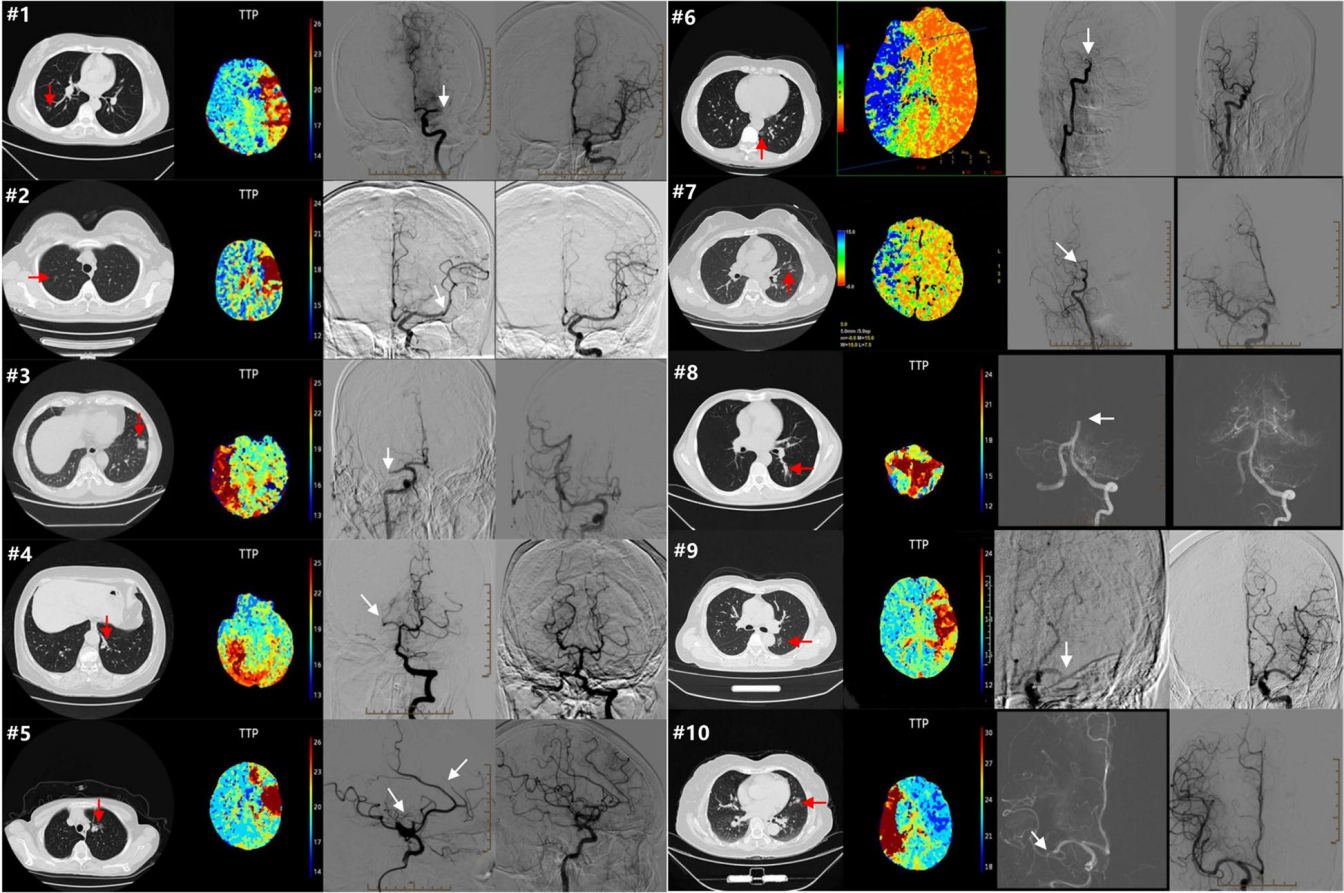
Medical images in 10 patients (location of lesion on Lung CT (red arrowheads), CT perfusion, location of the occlusive artery on DSA (white arrowheads))
